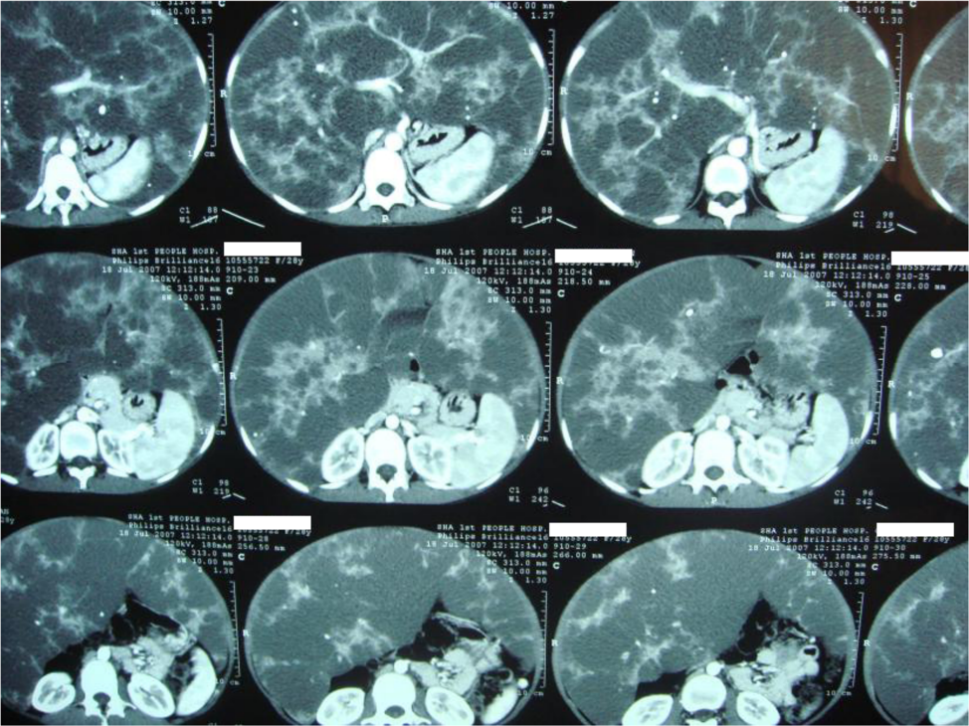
CT scan shows the giant hepatic hemangioma occupying the entire abdominal cavity.
